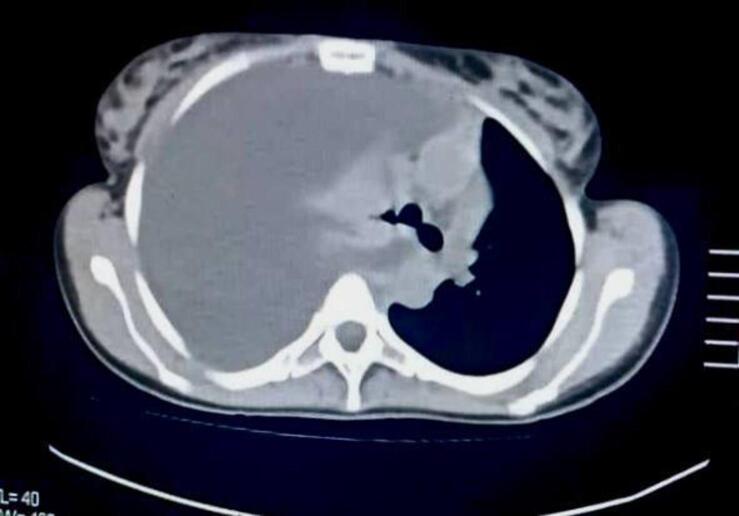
computed tomography (CT) which indicated a cavity likely containing a foreign body.
